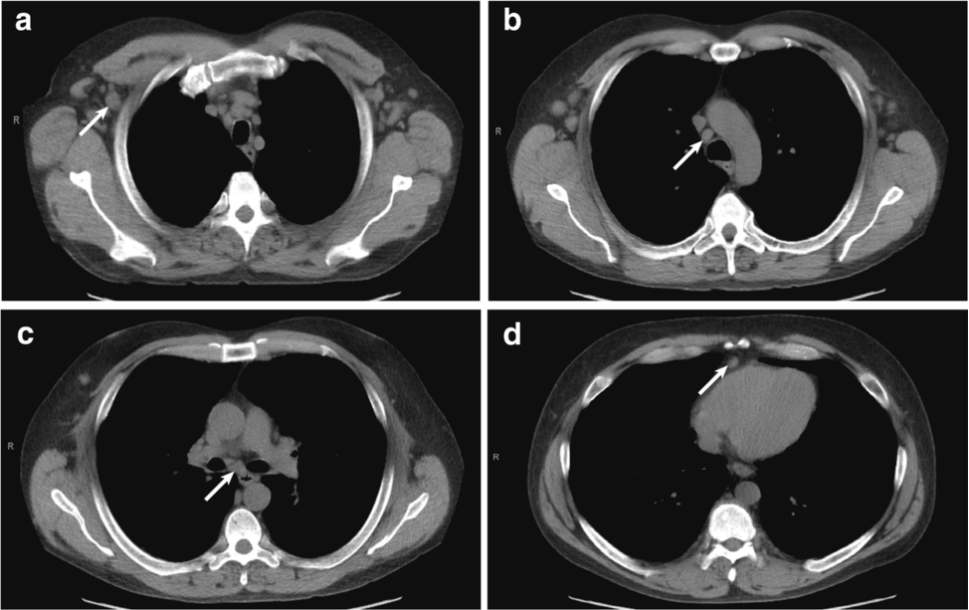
Lymphadenopathy on chest CT. Chest CT demonstrating enlarged lymph nodes (arrows) involving the right axillary (a), paratracheal (b), subcarinal (c), and cardiophrenic (d) regions.
